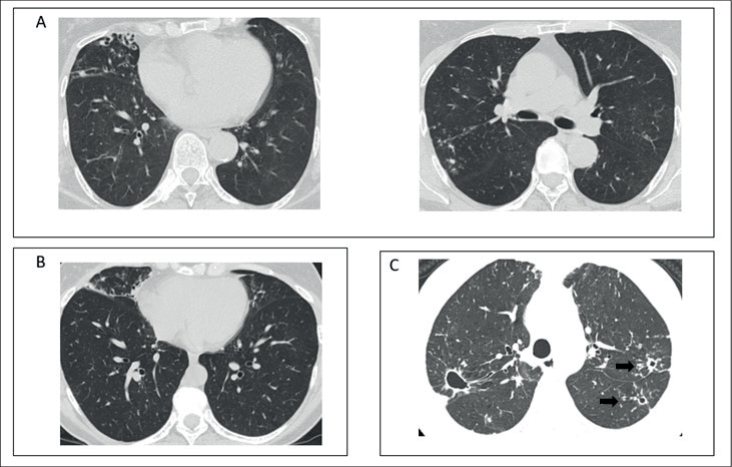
(A) CT scan of a patient with *M. fortuitum*; bronchiectasis in the middle lobe is associated with micronodules in the right upper lobe. (B) CT scan of a patient with *M. intracelulare;* bronchiectasis and micronodules is visible in the middle lobe and lingula. (C) Cavitation with thick walls in the upper lobes and centrilobular micronodules and some with “budding tree” pattern in the left upper lobe (black arrows).
